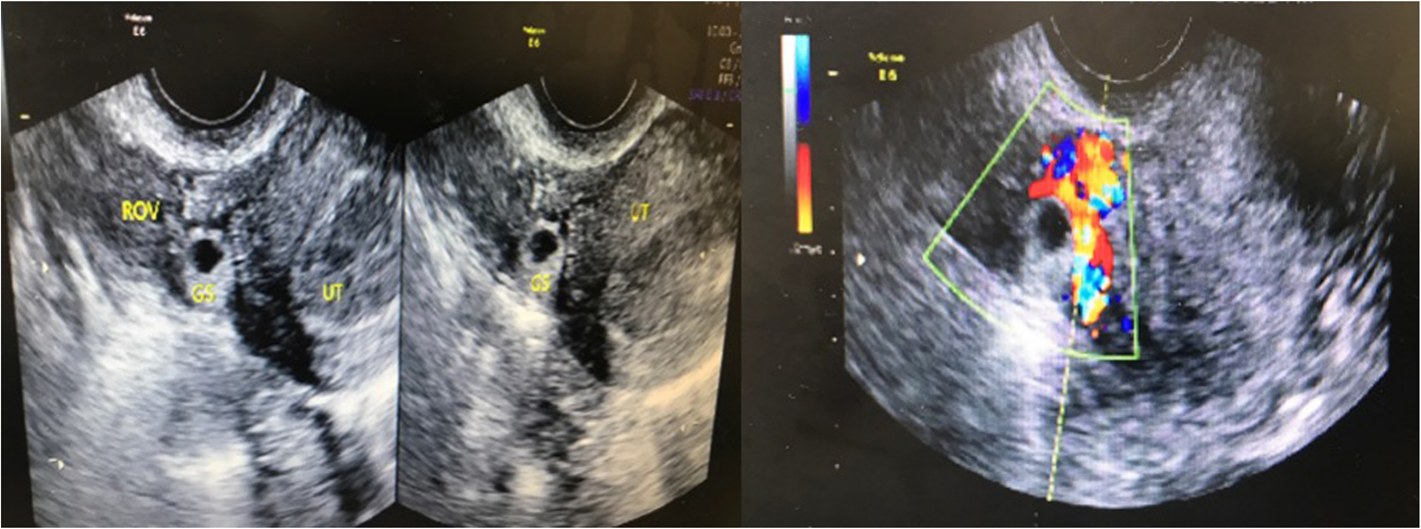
Transvaginal ultrasonography showed the gestation located between uterus and right ovary. Doppler flow parameters showed elevated peritrophoblastic blood flow
